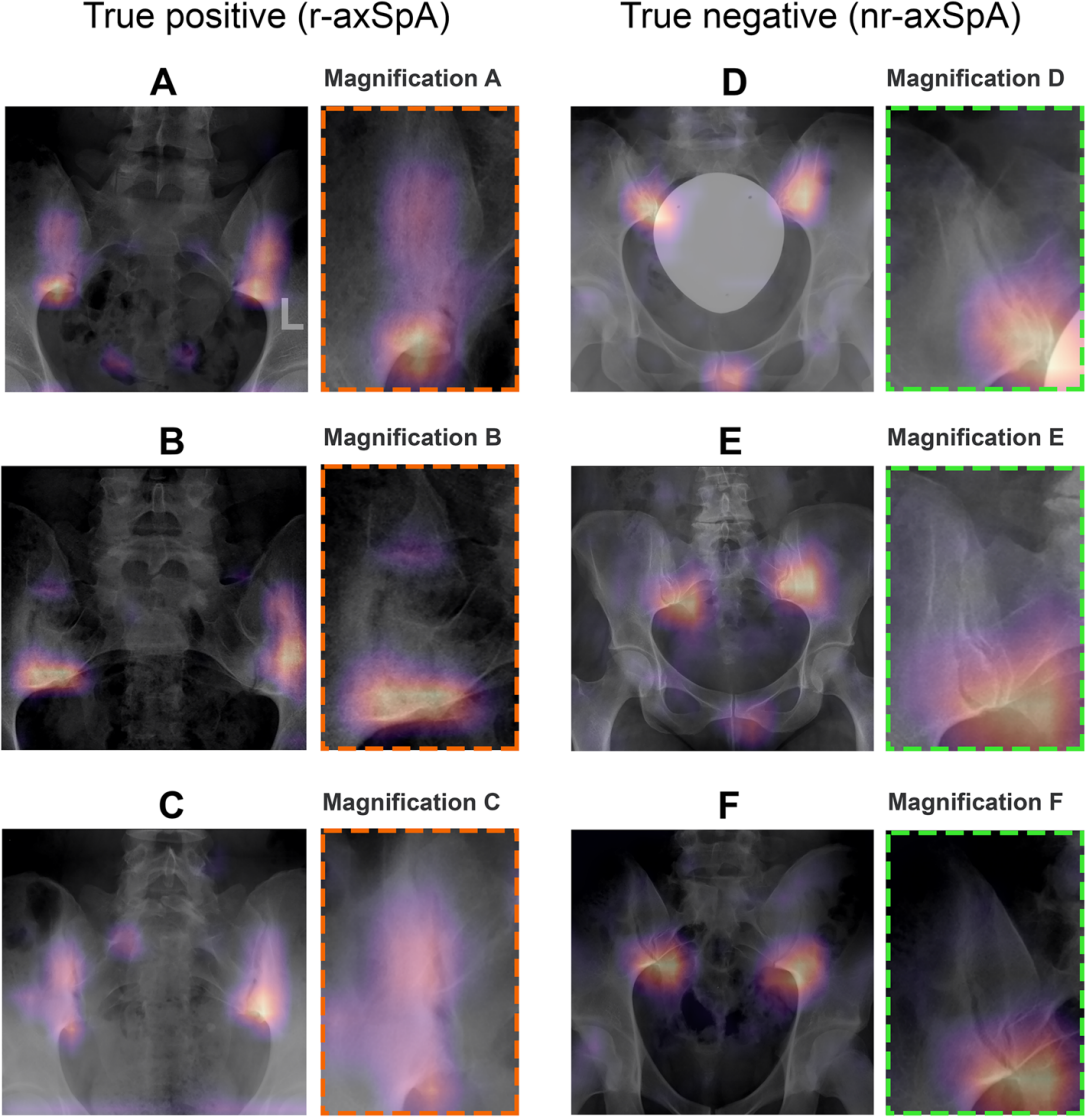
Gradient-weighted Class Activation Maps (Grad-CAMs) of the model for selected images taken from the test dataset. Grad-CAMs provide visual explanations for the model decision, as they highlight the important image regions that led to the model’s decision. The examples presented show that the model almost exclusively and correctly focusses on the sacroiliac joints to predict whether definite radiographic sacroiliitis is present or not
